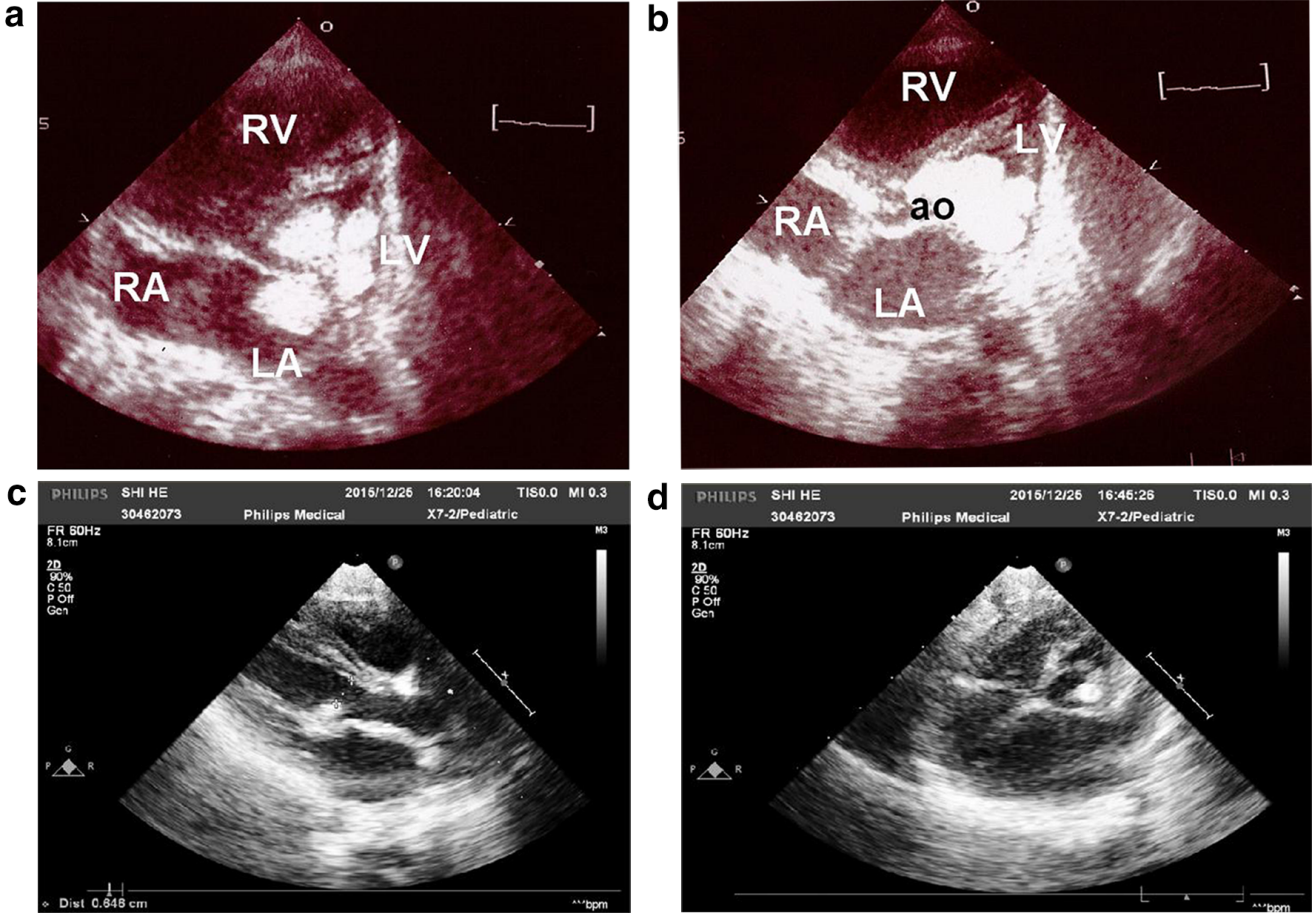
Case 2: a, b Echocardiogram on the second day of life, showing a 3-lobe LV tumor completely obstructed the mitral valve orifice and the subaortic area. c, d After 2 months of everolimus therapy, the LV tumor regressed to a small nodule attached at the lateral papillary muscle of the mitral valve. Both the mitral orifice and the subaortic area now have adequate room for blood flow
